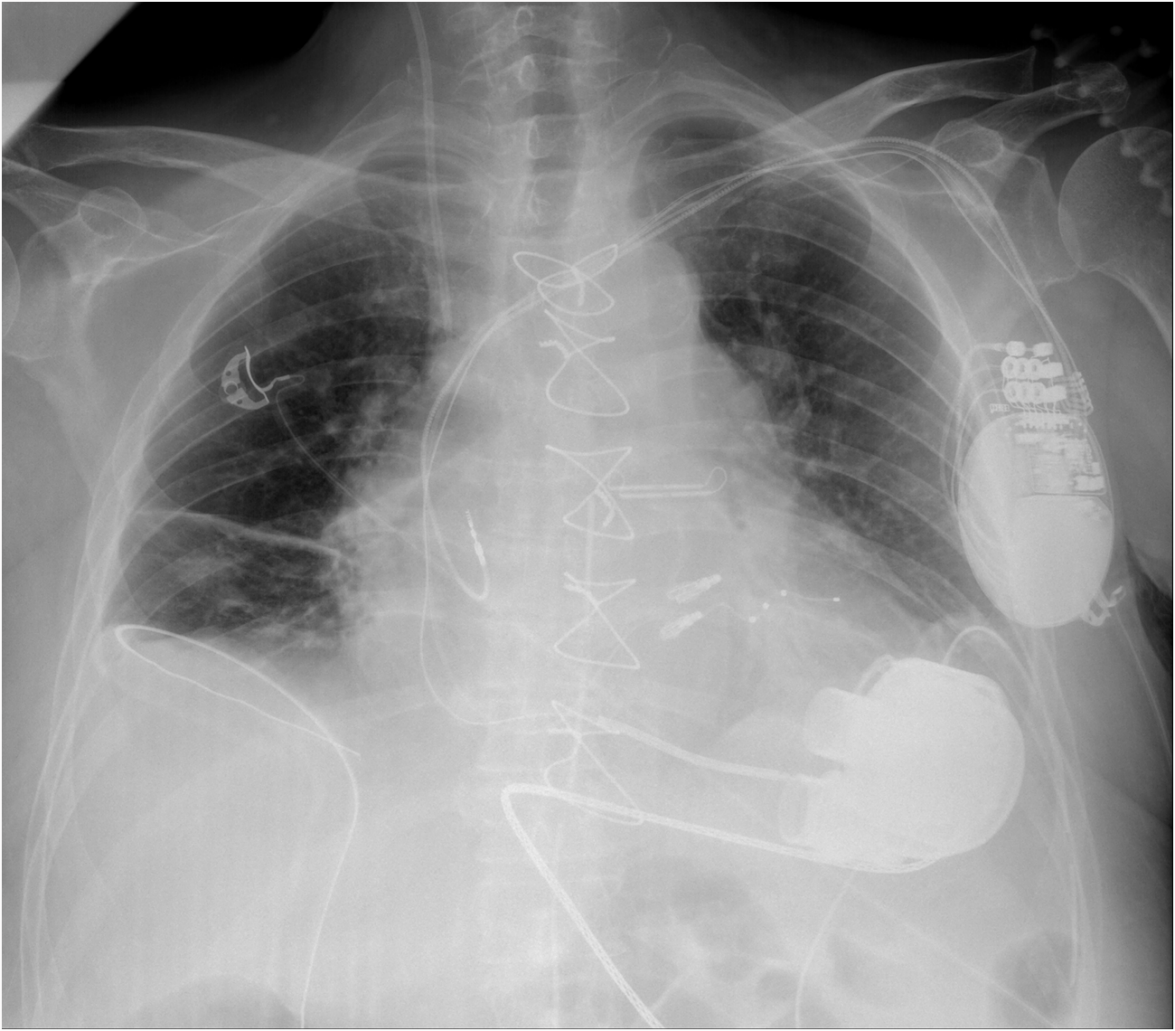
Chest X-ray showing the cardiac silhouette, CRT-D system leads and device, implanted two MitraClip clips, and the HeartMate 3 LVAD. CRT-D, cardiac resynchronization therapy with defibrillator; LVAD, left ventricular assist device
